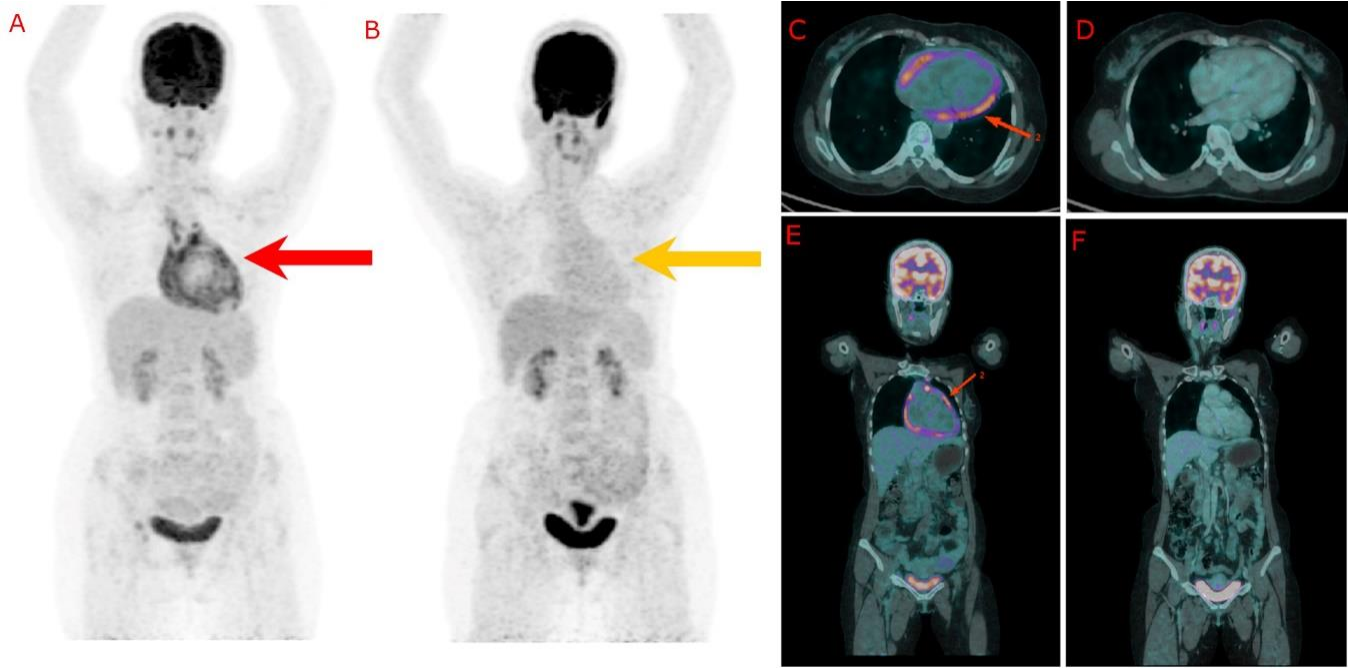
A: MIP whole body image before ATT showing pericardial FDG uptake (red arrow), B: MIP whole body image after ATT showing resolution of FDG uptake (yellow arrow), C: Fused axial image of the patient before ATT showing pericardial FDG uptake (red arrow) D: Fused axial image of the patient after ATT E: Fused coronal image of the patient showing pericardial FDG uptake (red arrow) F: Fused coronal image of the patient after ATT showing resolution of FDG uptake. MIP - Maximum intensity projection. ATT - Anti-tubercular therapy, FDG - Fluorodeoxyglucose
